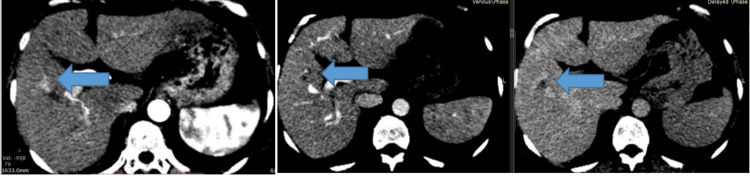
Axial sections of CT triphasic of liver: small area of arterial enhancement in segment 5 with washout on venous and delayed phase concerning for hepatocellular carcinoma.
